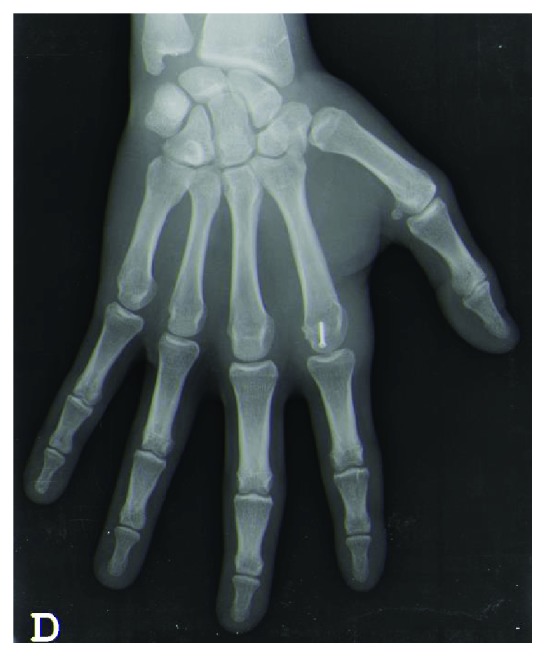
X-Ray (AP view) showing fracture consolidation at week 6.
